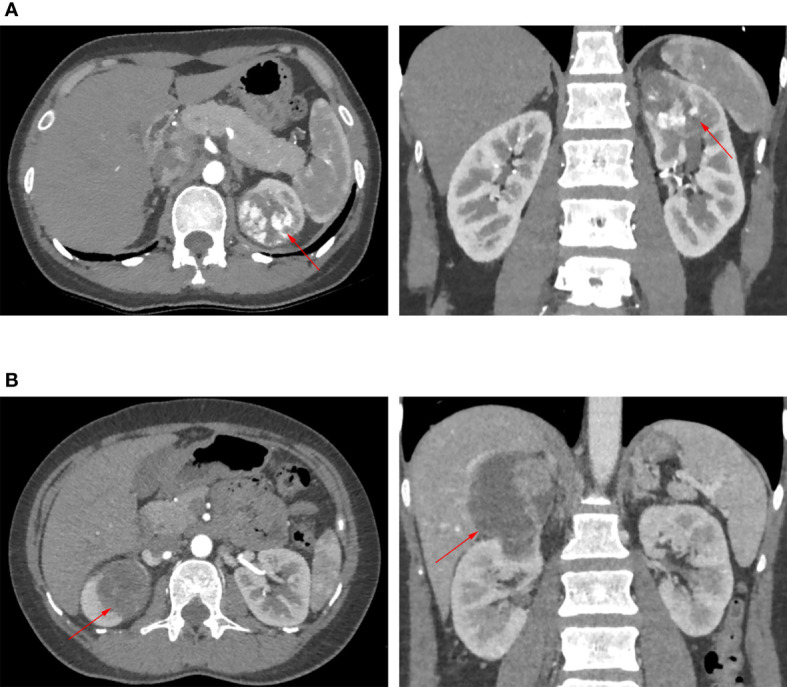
Representative images of CT or MRI. Red arrow points to renal tumor. (A) The patient underwent transperitoneal robotic partial nephrectomy. (B) The patient underwent retroperitoneal robotic partial nephrectomy.
